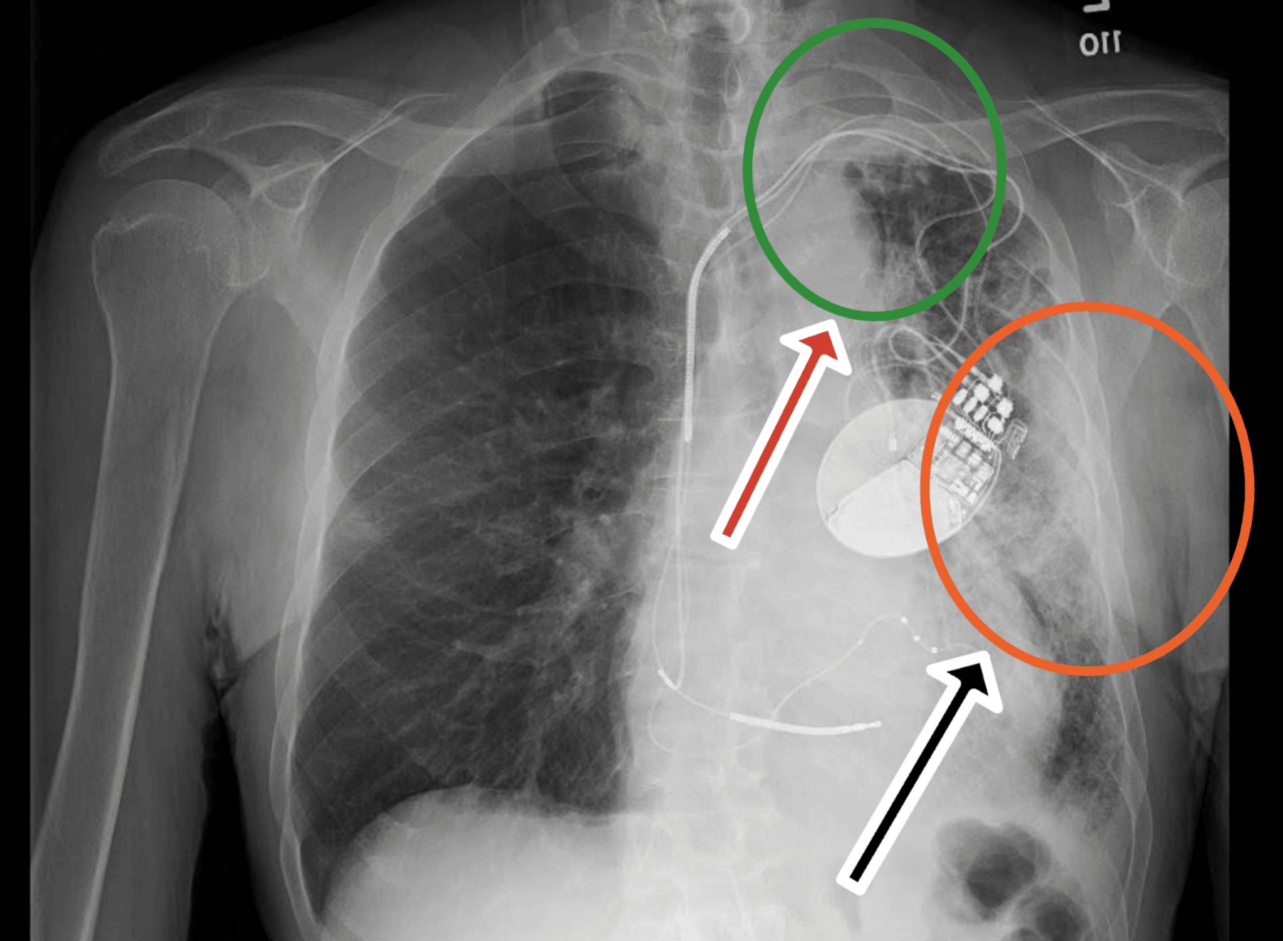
Chest X-ray showing new interstitial thickening and patchy airspace opacities confined to the left upper (red arrow) and lower lobes (black arrow). The marked unilateral distribution is atypical for amiodarone-induced pulmonary toxicity, which more commonly presents with bilateral involvement, and raises diagnostic consideration for alternative focal processes such as infection or malignancy.
